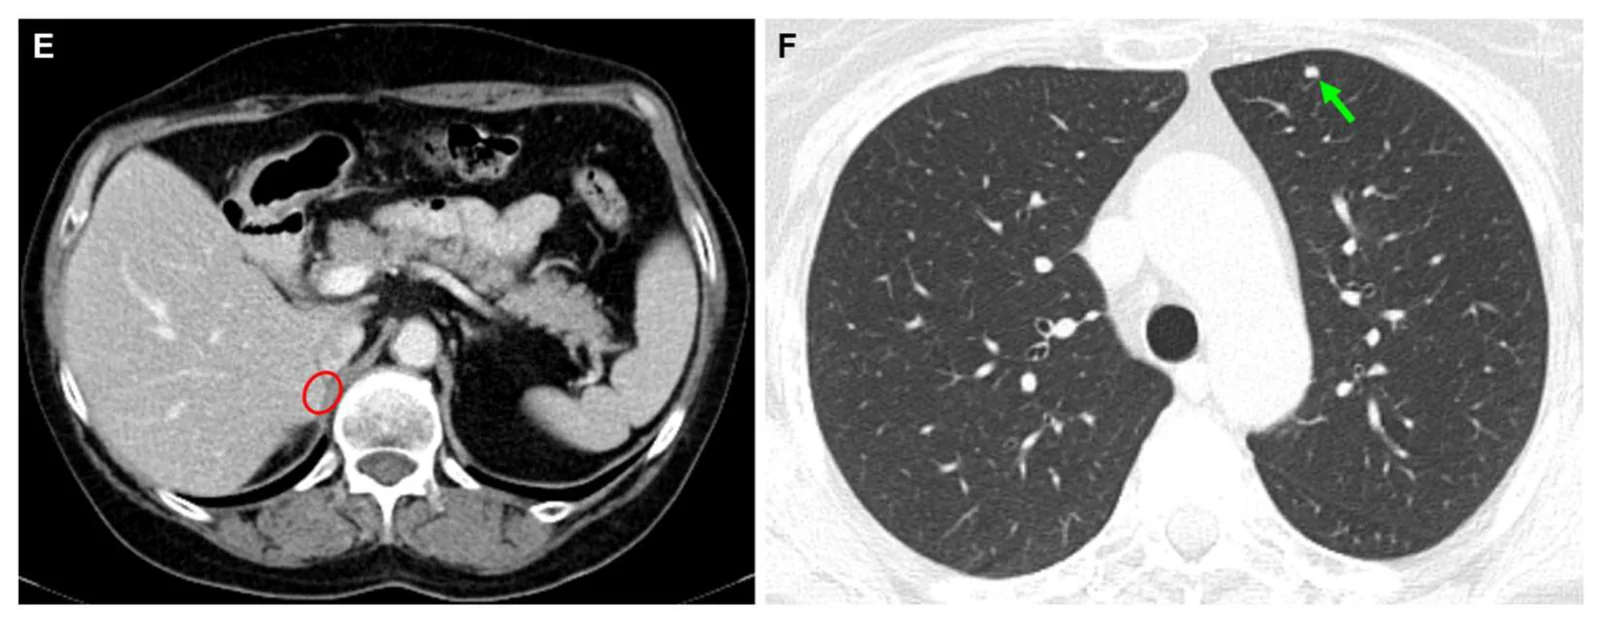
The patient underwent a 5-year protocol of mitotane (between 2012 and 2017), which she tolerated well. In the meantime, CT scans showed no local recurrence in the adrenal area and two stationary pulmonary micronodules. The adrenal failure was well controlled with 5 to 7.5 mg/day of oral prednisone and 0.1 mg/day of fludrocortisone. She developed primary hypothyroidism requiring daily oral levothyroxine therapy (50 µg/day and later 75 µg/day) and showed a tendency to ecchymoses and easy bruising (Figure S2). [CT scan in the year 2015 (A,B): (A) right adrenalectomy area with postsurgical fibrosis (red circle), no image of local tumoral recurrence (contrast-enhanced CT scan, axial plane, venous phase); (B) pulmonary nodule of 5.1 mm (green arrow) in the anterior segment of the left upper lobe (contrast-enhanced CT scan, axial plane, lung window); CT assessment in the year 2016 (C): (C) pulmonary nodule of 4.5 mm (yellow arrow) in the apico-posterior segment of the left upper lobe (contrast-enhanced CT scan, axial plane, lung window); CT scan in the year 2017 (D–F): (D) pulmonary micronodule of 6 mm (yellow arrow) in the apico-posterior segment of the left upper lobe (contrast-enhanced CT scan, axial plane, lung window); (E) right adrenalectomy area with postsurgical fibrosis (red circle), no image of local tumoral recurrence (contrast-enhanced CT scan, axial plane, venous phase); (F) pulmonary nodule of 5.1 mm (green arrow) in the anterior segment of the left upper lobe (contrast-enhanced CT scan, axial plane, lung window)].
